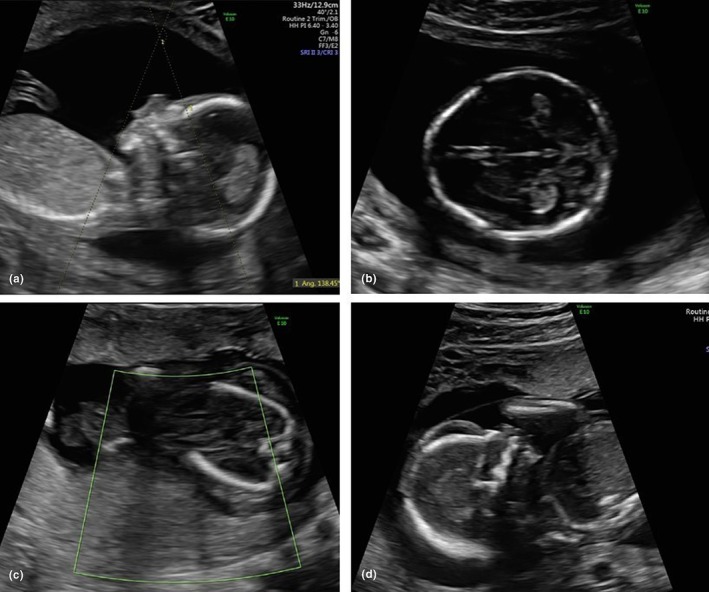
(a, b) Early anomaly scan at 16^+2^ weeks of gestation: facial profile showing micrognathia with an inferior facial angle of 42° and trigonocephaly. (c, d) Ultrasound at 19^+3^ weeks of gestation: absence of cardiac activity on color Doppler and hydrops of the chest and abdomen, generalized hydrops also involving the face.
